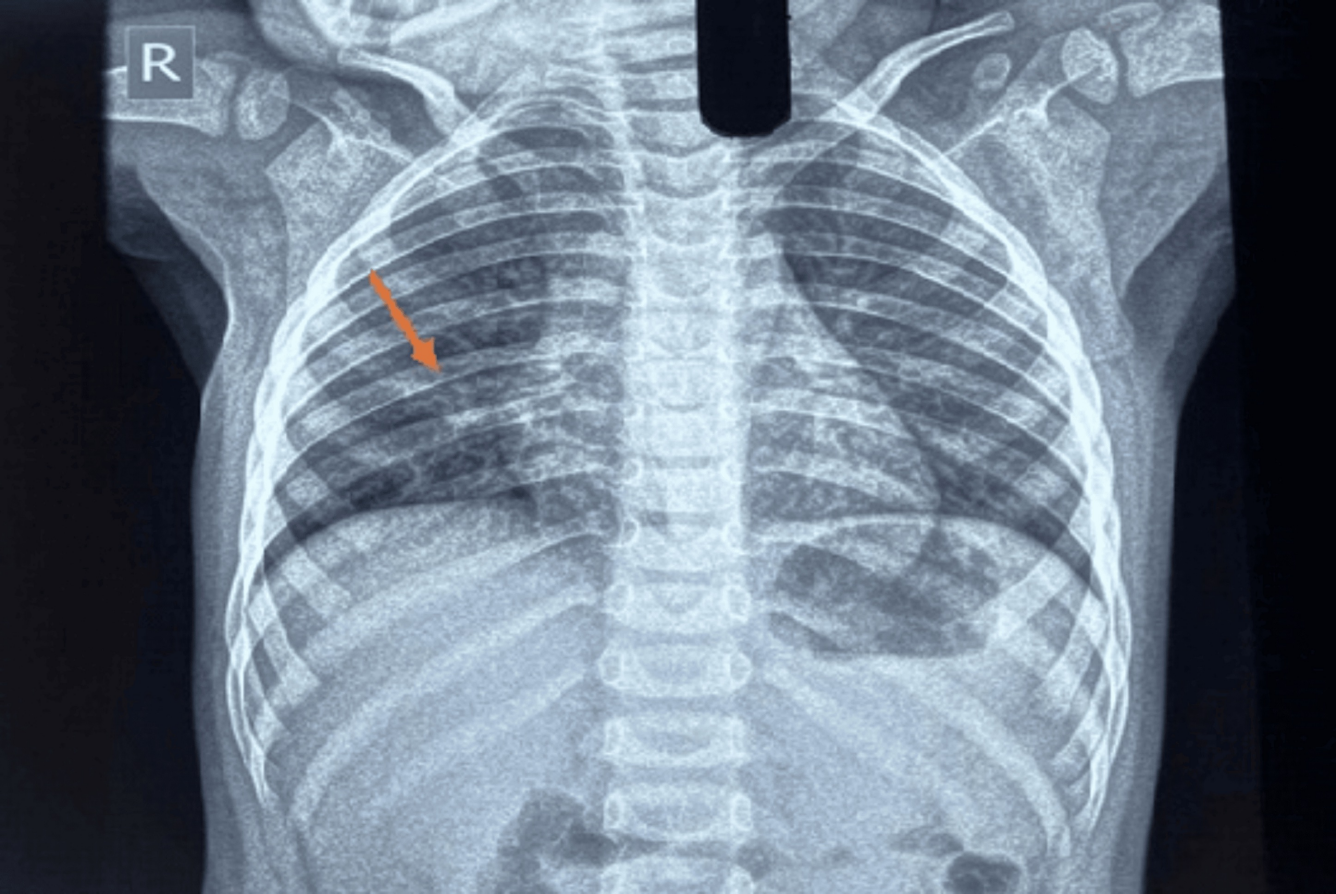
Chest X-ray Bilateral patchy perihilar and lower-zone air-space opacities with prominent bronchovascular markings suggestive of pneumonitis.
